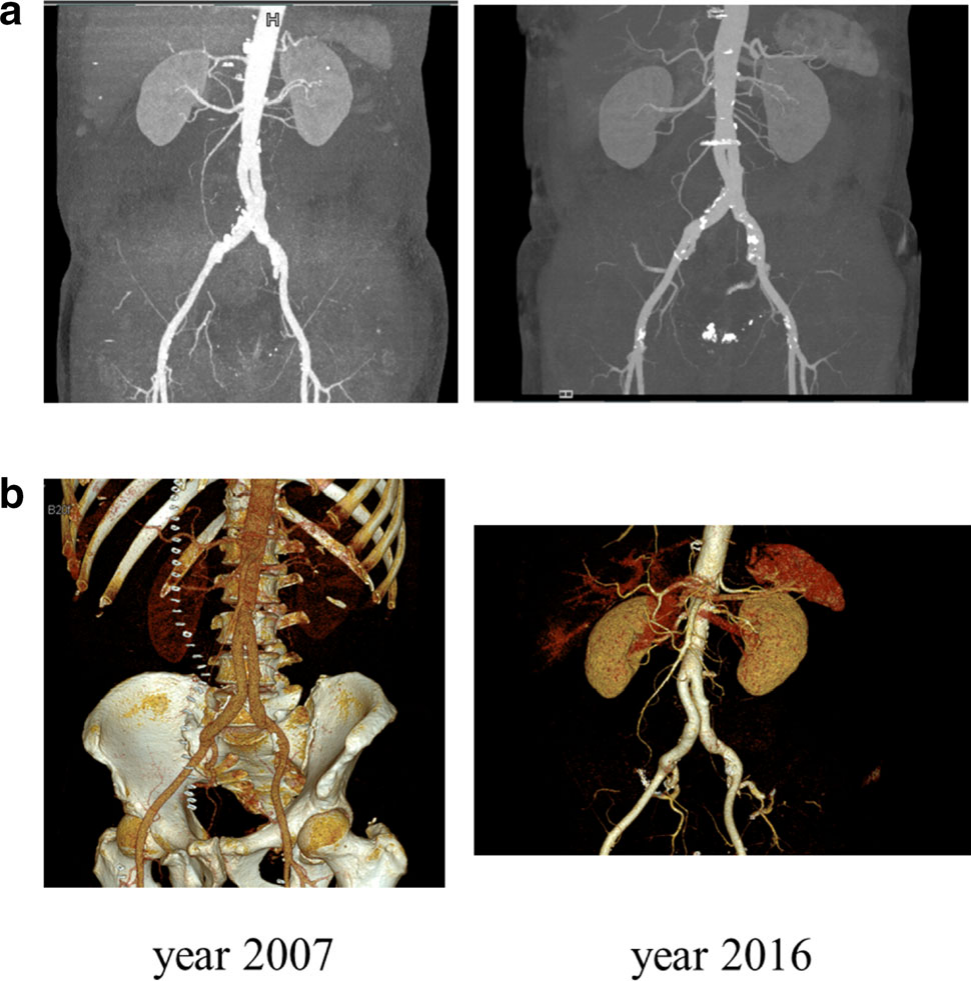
Bifurcational “pantalon” FV graft [17]. Postoperative CTA scan with three-dimensional reconstruction after 1-month a and 9 years b after surgery. Again, proximal as well as distal anastomoses are significantly elongated; however, the bypass is patent
